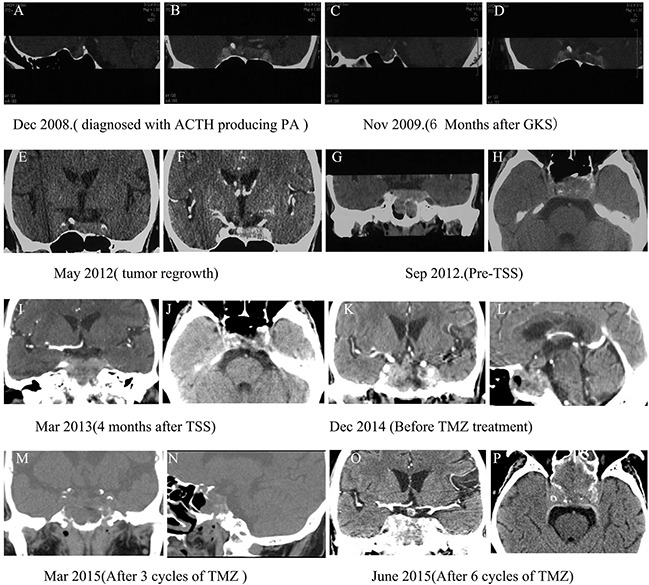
Computed Tomographic (CT) images of pituitary tumors in the case two patient A and B. CT images at initial diagnosis. C and D. Six months after GKS. E and F. CT scan from May 2012 showing significant tumor progression. G and H. CT scans from September 2012 showing significantly enlarged pituitary masses that invaded the sphenoid and cavernous sinuses. I and J. CT scan 4 months after TSS showing residual tumor regrowth. K and L. Before TMZ administration. M and N. After 3 cycles of TMZ. O and P. After 6 cycles of TMZ.
